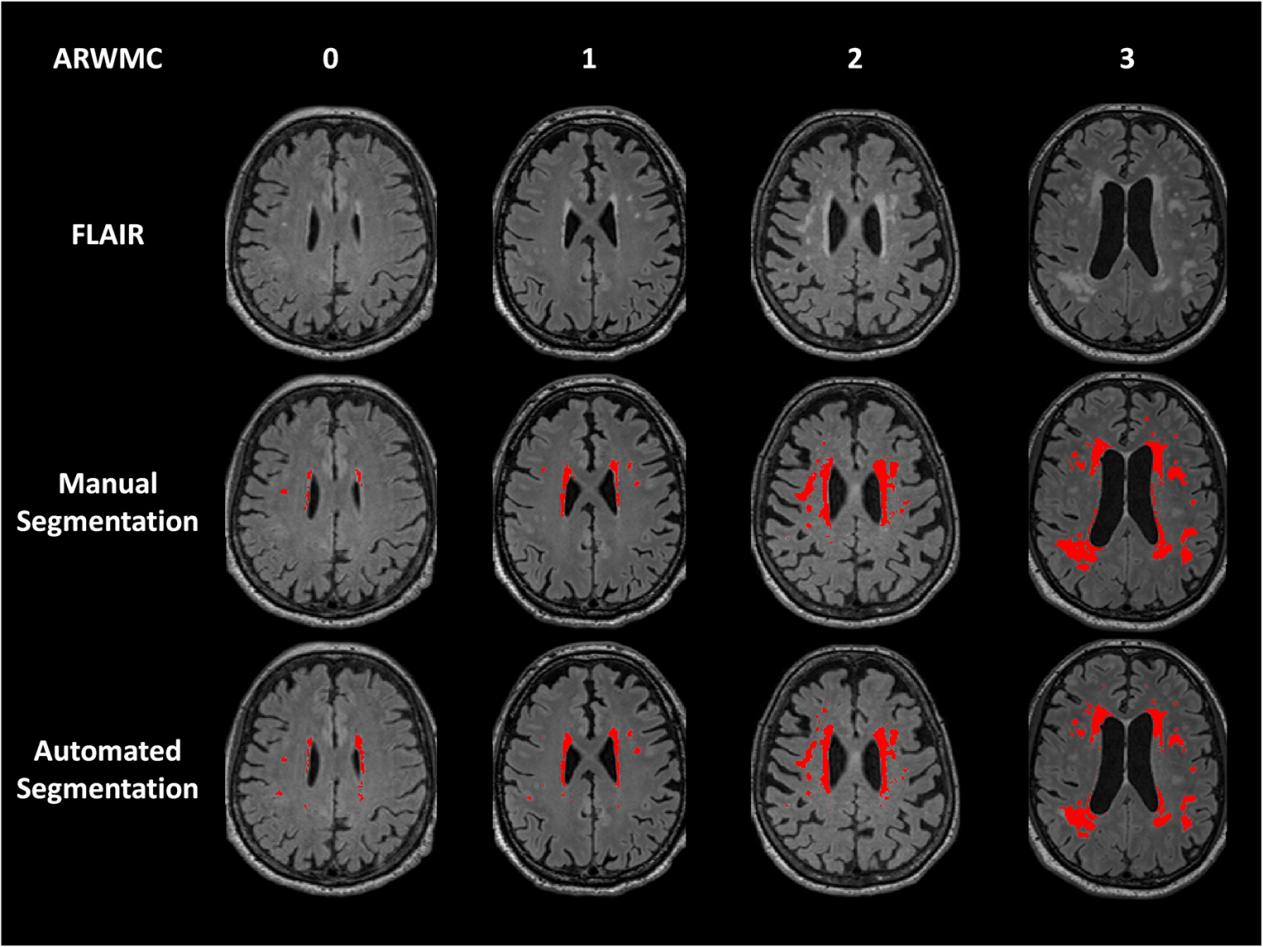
Examples of manual and automated segmentation of white matter hyperintense lesions according to age-related white matter changes score (ARWMC) on FLAIR images
